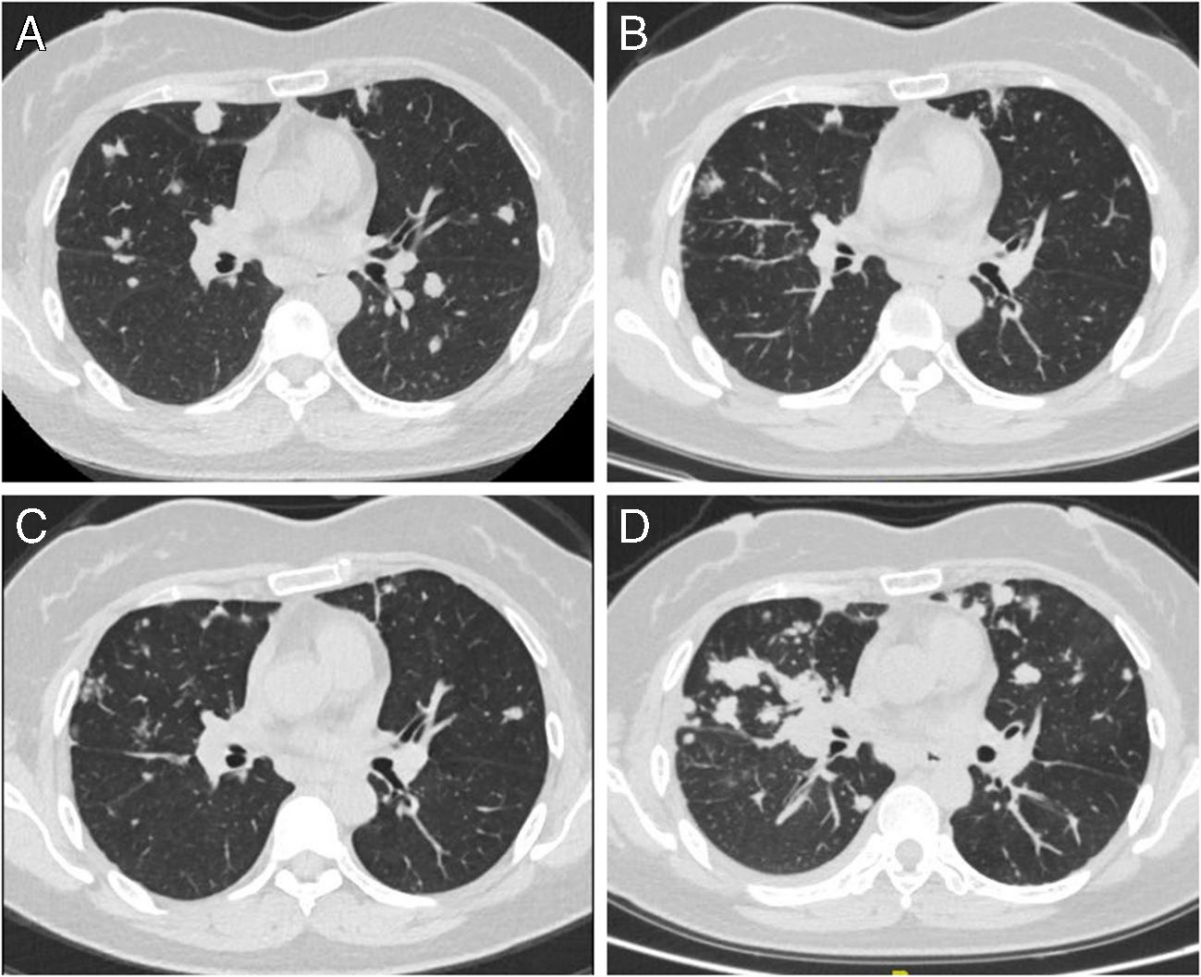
Chest computed tomography (CT) demonstrated lung metastases before surufatinib treatment (A, July 22, 2021), on the second month of surufatinib treatment (B, September 23, 2021; the tumour size was reduced), on the fourth month of surufatinib treatment (C, November 17, 2021; the tumour size was not further reduced), and after surufatinib discontinuation (D, February 8, 2022, 49 days from the last dose)
